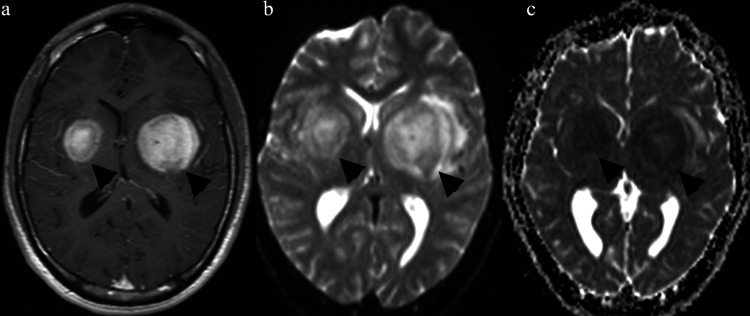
MRI (a) Axial contrast-enhanced T1-weighted magnetic resonance images showing lesions in the basal ganglia, larger on the left, with lateral ventricle compression (black arrowheads); (b) the same on the diffused-weight imaging map with signal restriction and high signal strength (black arrowheads), and (c) on the apparent diffusion coefficient map with low signal intensity (black arrowheads)
